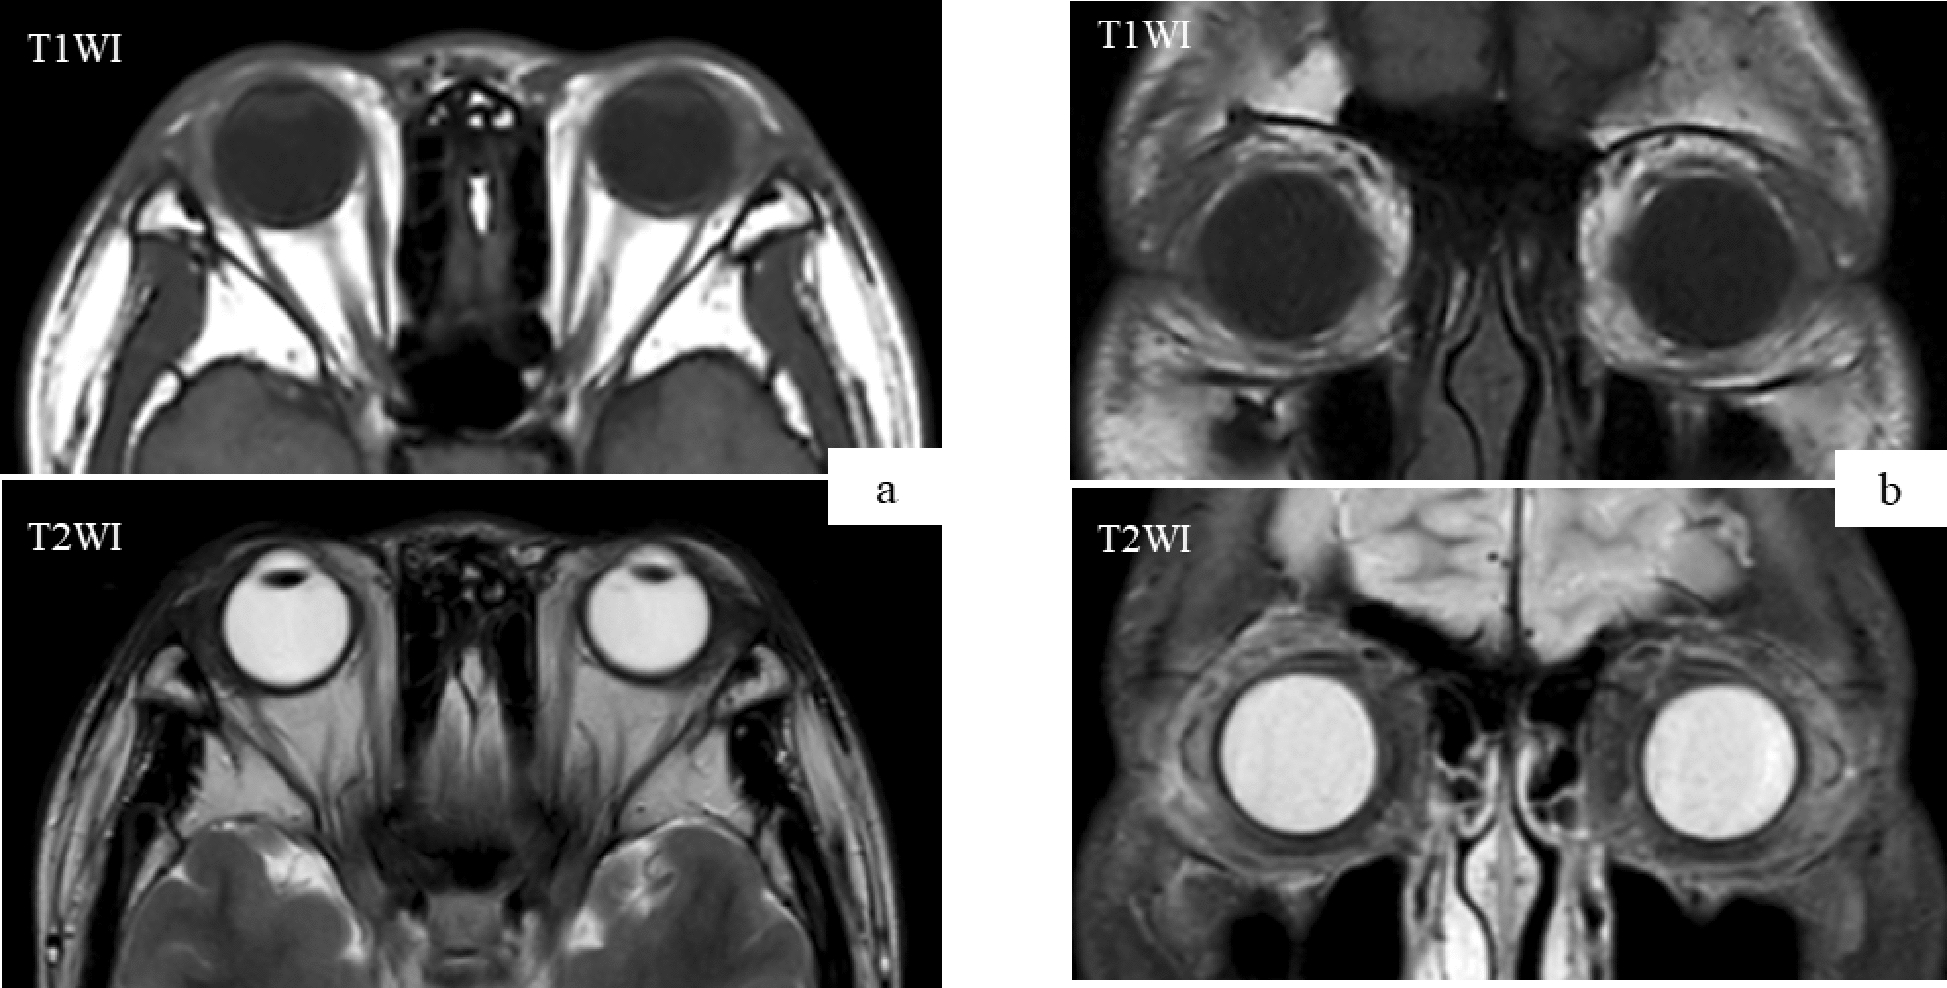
Magnetic resonance imaging (after second operation). a, b Axial and coronal MRI demonstrated no presence of an enhanced lesion of the bilateral lacrimal gland
